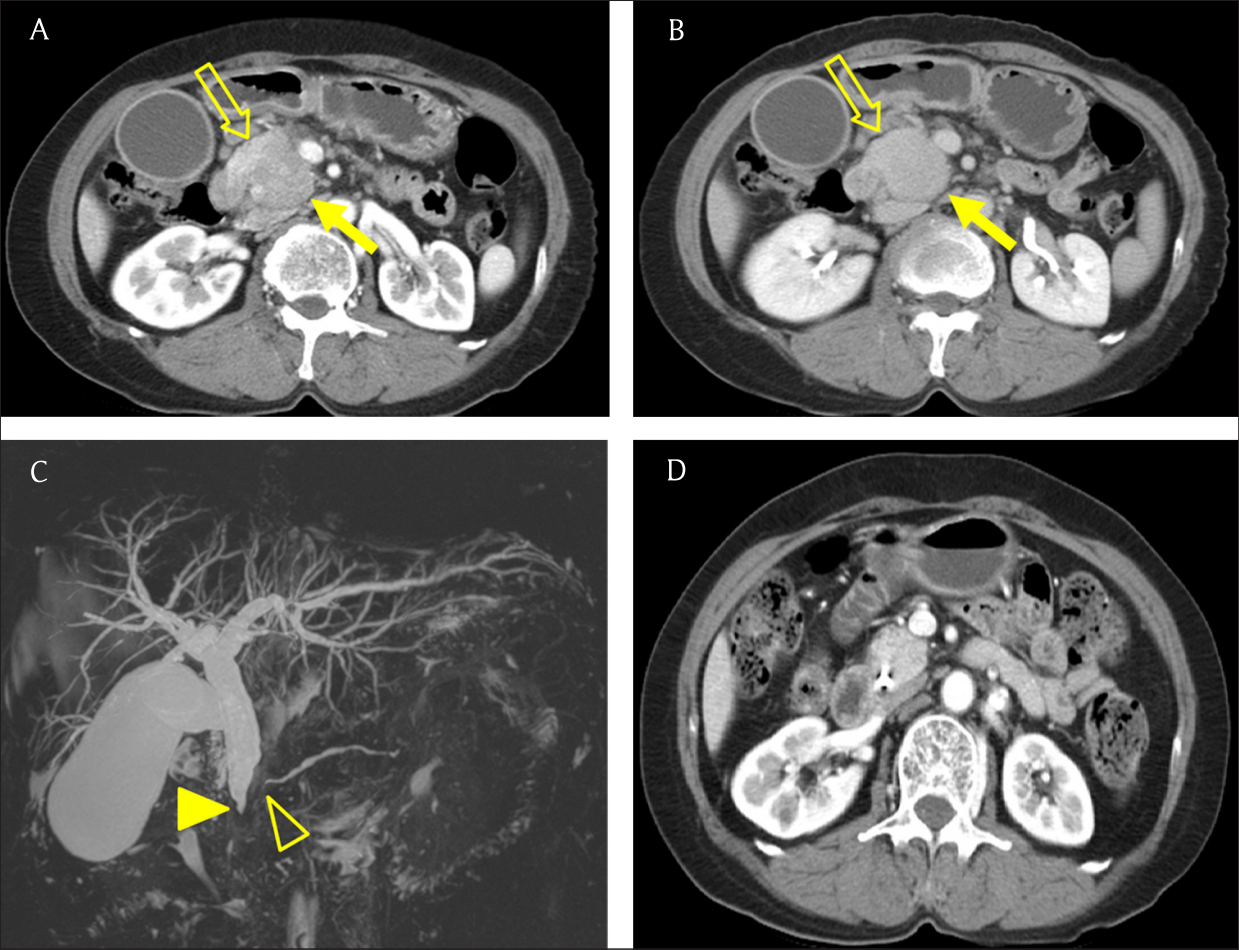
A 67-year-old woman with focal type autoimmune pancreatitis. (A) Axial pancreatic phase CT image shows a low attenuating mass (arrow) in the pancreatic head. Note the relatively high attenuation of the normal pancreatic parenchyma (open arrow). (B) On axial portal venous phase CT image, the mass (arrow) shows delayed enhancement and similar attenuation to the normal pancreas parenchyma (open arrow). (C) MRCP image shows dilatation of the bile and pancreatic ducts with smooth tapered narrowing (arrowhead and open arrowhead). (D) On axial portal venous phase CT image obtained after 50 days, the pancreatic lesion has resolved.
